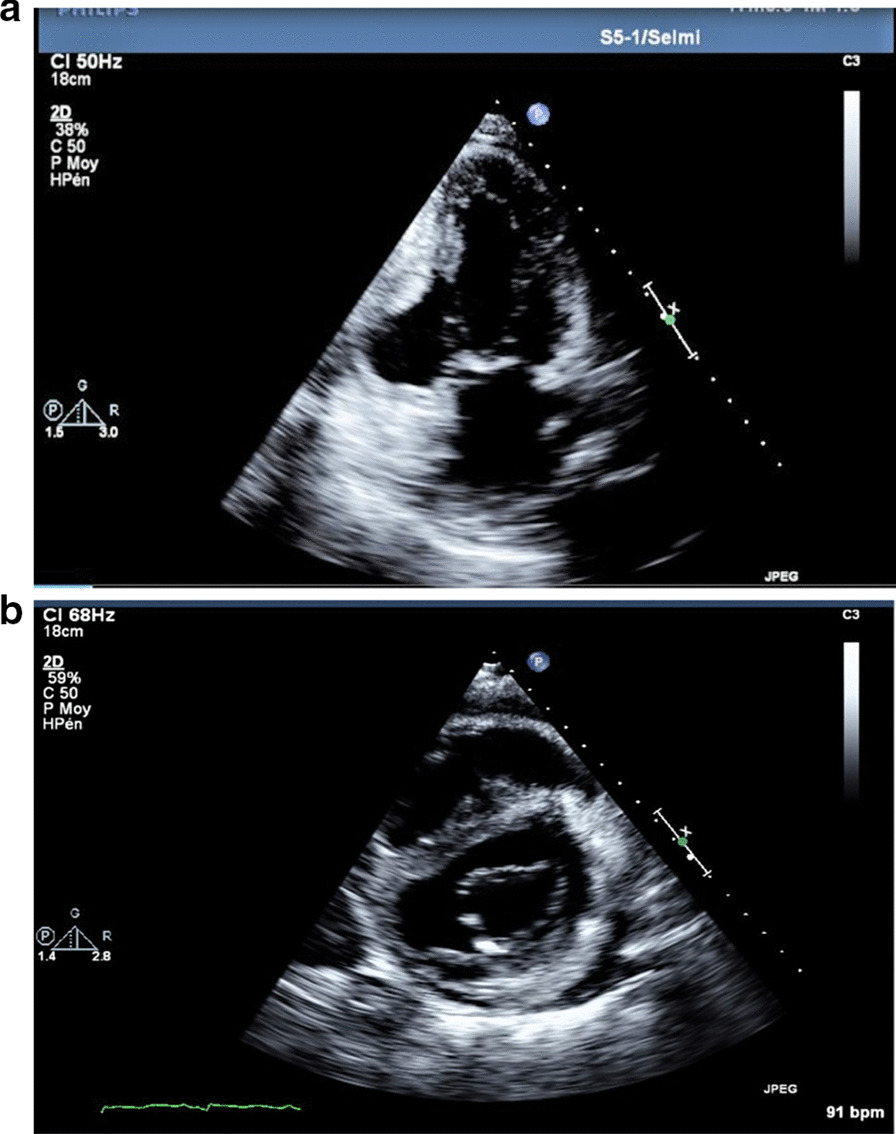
a Transthoracic echocardiogram from apical two-chamber view showing a large pseudoaneurysm from the inferior and inferolateral wall of the left ventricle. b Transthoracic echocardiogram from parasternal short axis view showing a large pseudoaneurysm from the inferior and inferolateral wall of the left ventricle
